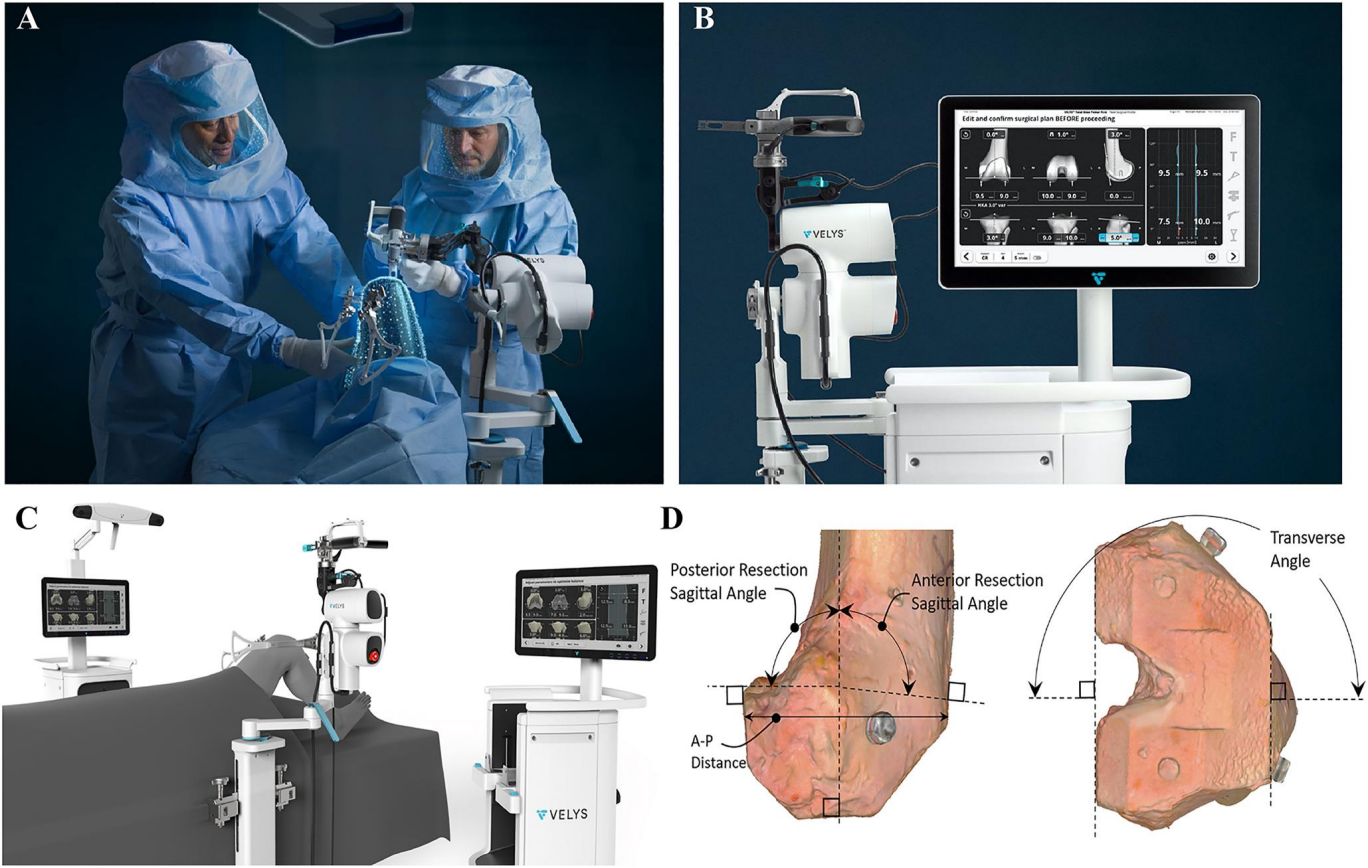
(A) VELYS™ is used in total knee arthroplasty. (B) Software systems for evaluation and forecasting. Reproduced with permission.^36^ Copyright 2022, DePuy Synthes. (C) VELYS™ includes an optical tracking system, a positioning needle and a robotic arm that is positioned at the head of the surgical bed. (D) Resection models produced by CT in the sagittal and cross‐sectional planes of the femur. Reproduced under terms of the CC‐BY license.^38^ Copyright 2022, The Authors, published by Elsevier.
